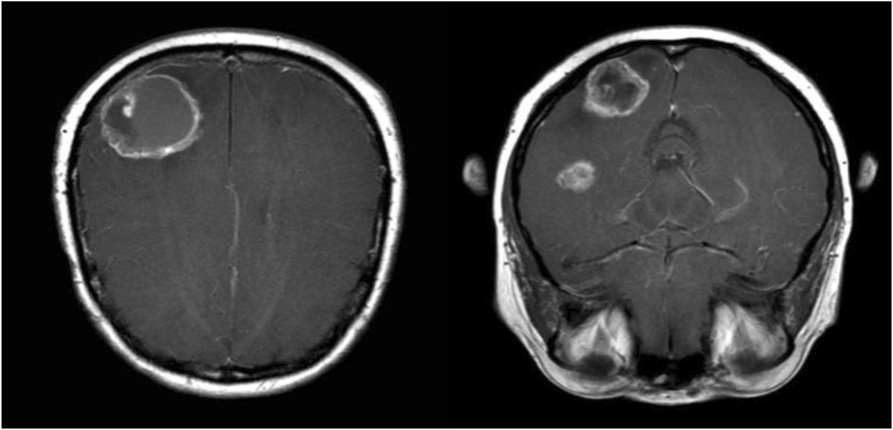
Gadolinium-enhanced axial T1-weighted brain magnetic resonance imaging scans showing three metastatic cystic brain lesions in the right parieto-occipital and temporal regions with associated perifocal edema.
